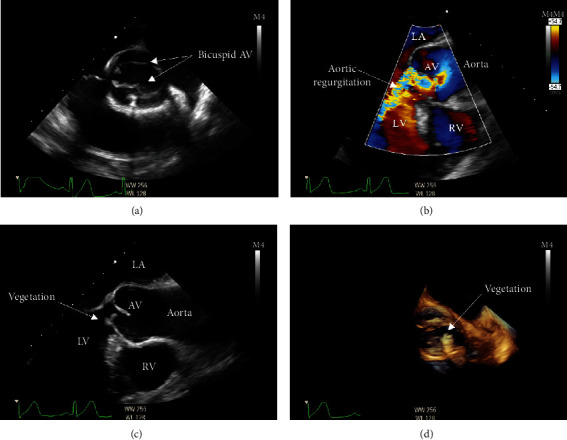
Transoesophageal echocardiogram images suggestive of bicuspid native aortic valve infective endocarditis. AV: aortic valve; LV: left ventricle; RV: right ventricle; LA: left atrium. Transoesophageal echocardiogram demonstrates the classical “fish mouth” appearance of aortic bicuspid valve during systole (a), severe aortic regurgitation (b), 5 × 7 mm vegetation adherent to the ventricular aspect of the aortic valve seen in two-dimensional echocardiogram (c), and three-dimensional echocardiogram (d).
